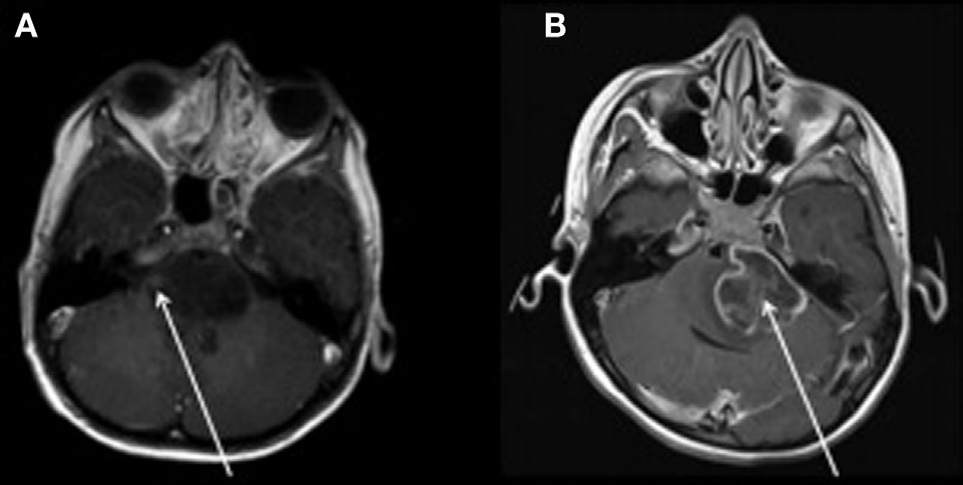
MRI examinations of two patients with diffuse intrinsic pontine gliomas (see arrows). (A) demonstrates a small nodular enhancement (arrow) and (B) demonstrates a large ring enhancement (arrow). Figure reproduced with permission (38).
